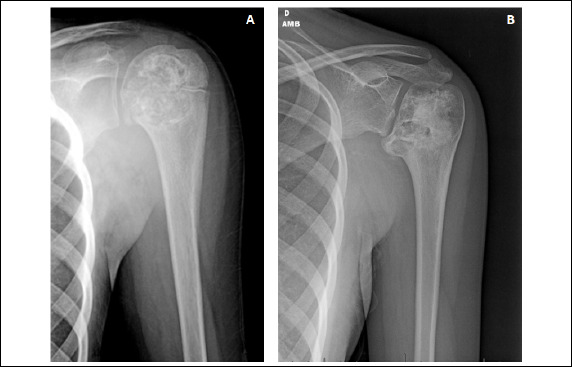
Shoulder X-ray of a patient with chondroblastoma Caption: A: Anteroposterior radiograph of the left shoulder of a 13-year-old girl with a history of moderate pain for a year, severe limitation of range of motion, with flexion and abduction of 30º. The bone lesion lies in the epiphysis of the proximal humerus, measuring 3.8 cm, occupying 74.0% of the epiphysis, and showing an eccentric shape, calcifications, and damaged partially closed physis. Surgical treatment was performed with curettage with a mechanical and thermal adjuvant and filling with an autologous graft of the iliac crest. B: Anteroposterior radiograph of the left shoulder four years after surgery. This study observed an important deformity of the humeral head, associated with deformity of the inferior surface of the glenoid, classified as Kellgreen-Lawrence 4.
